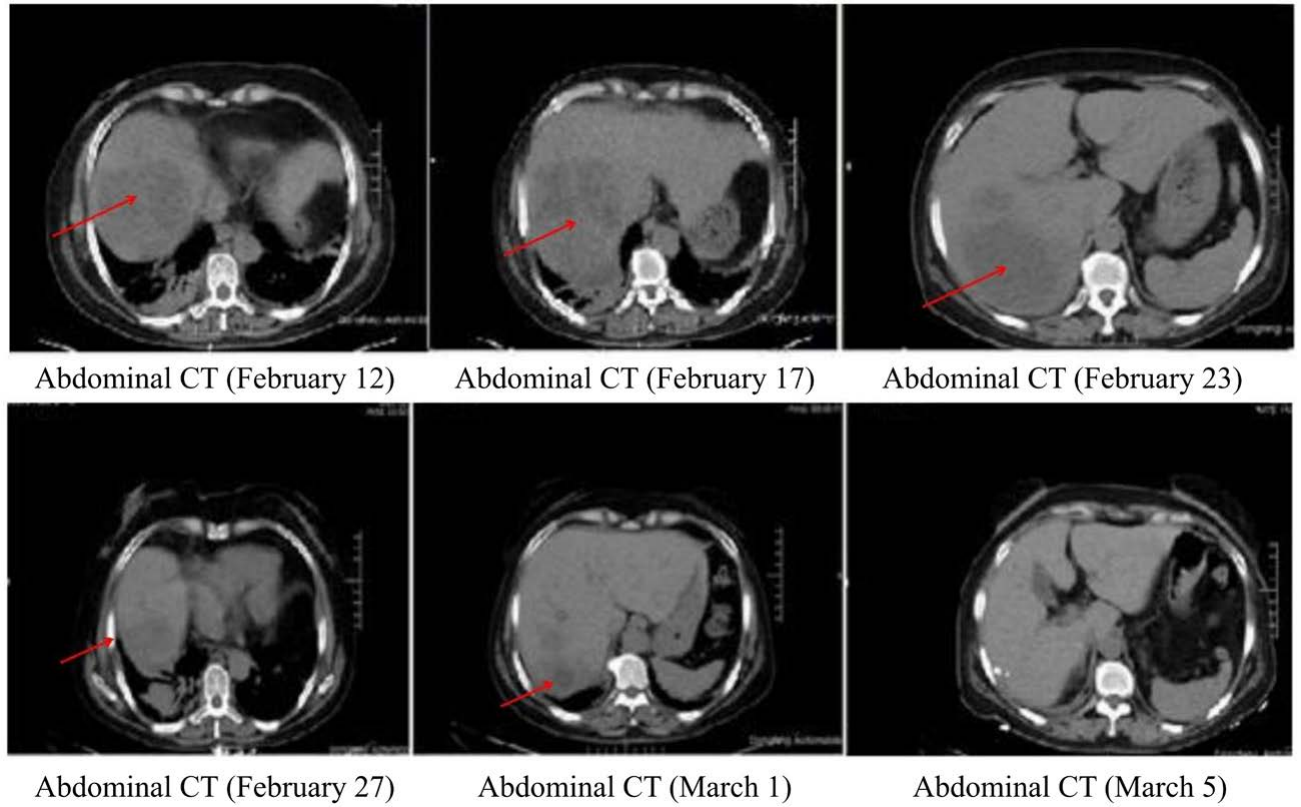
Abdominal CT. CT = computed tomography.
